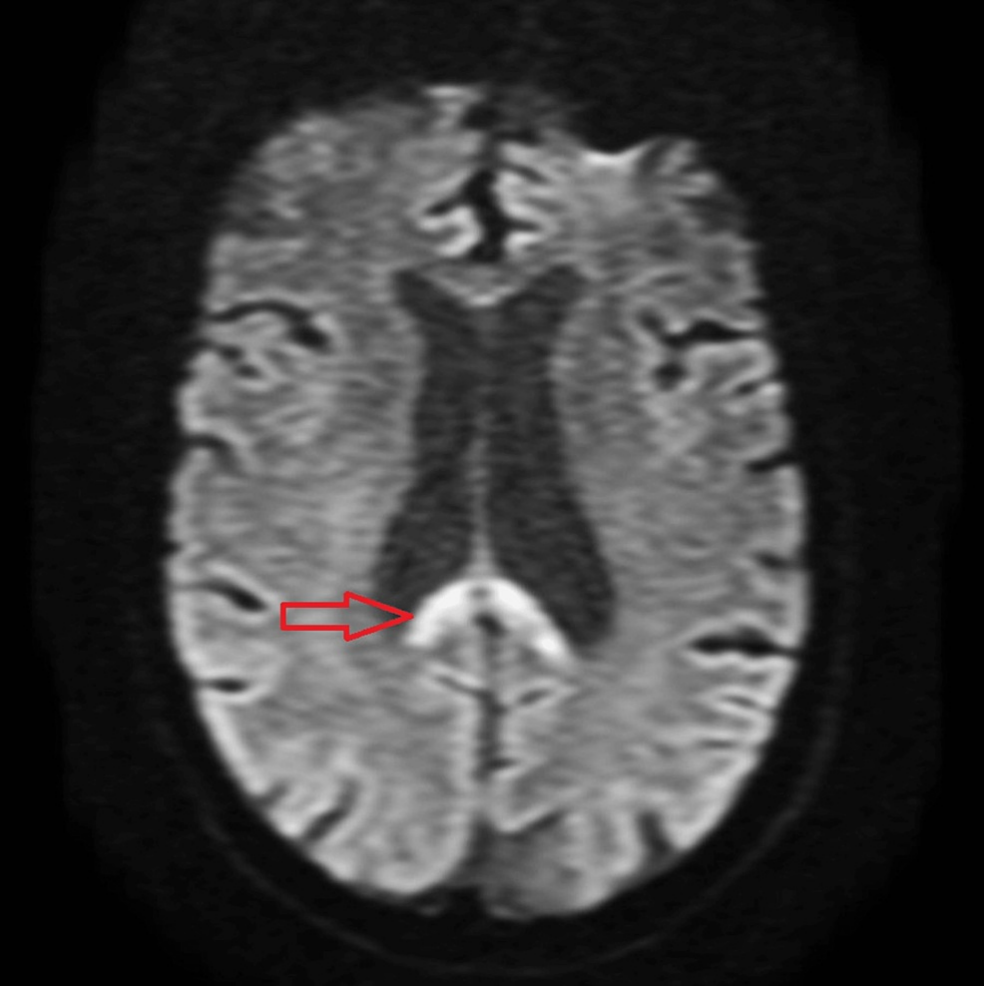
Axial DWI six months after the first MRI showing the attenuation of the restricted diffusion (arrow) compared to the initial MRI (Figure 3). DWI: diffusion-weighted imaging; MRI: magnetic resonance imaging
